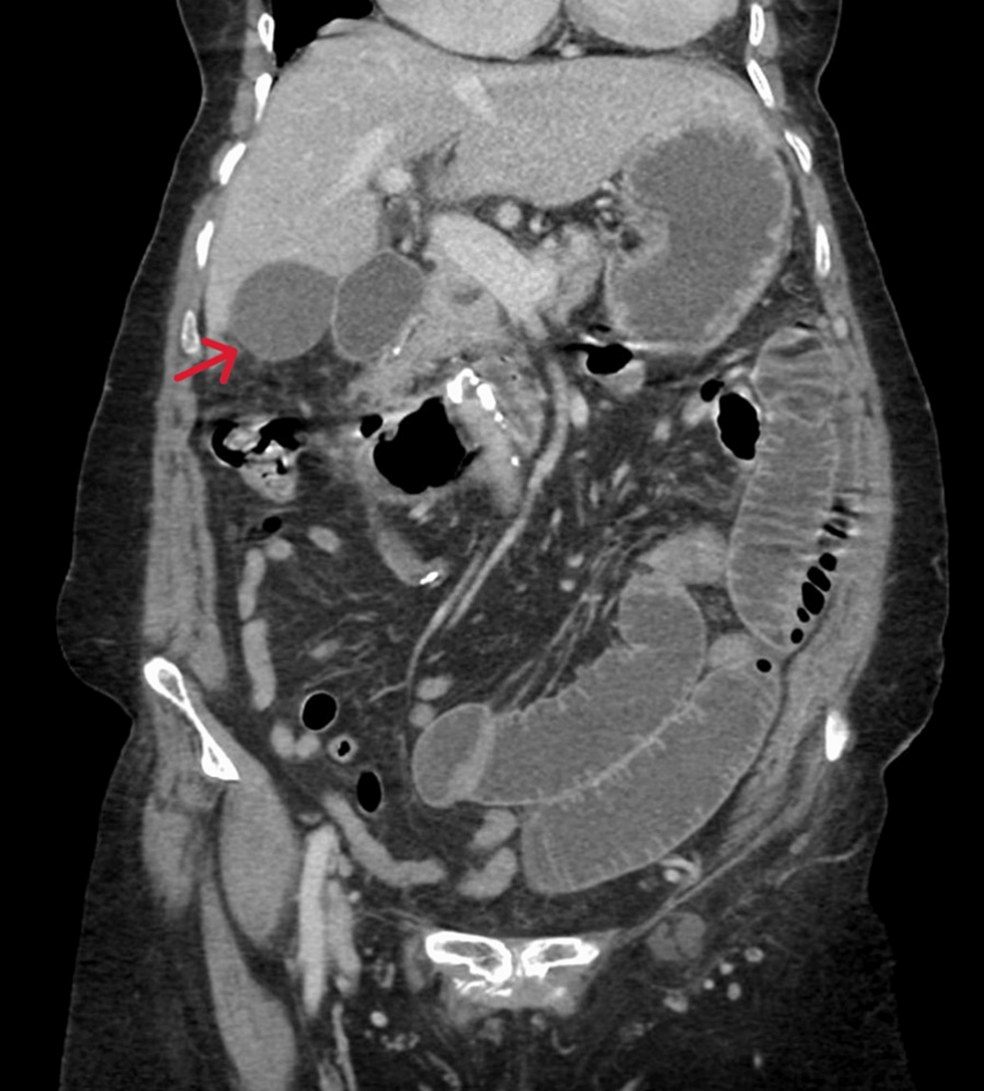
Abdominal CT in coronal view showing right subhepatic space cystic structure (red arrow) CT: computed tomography
